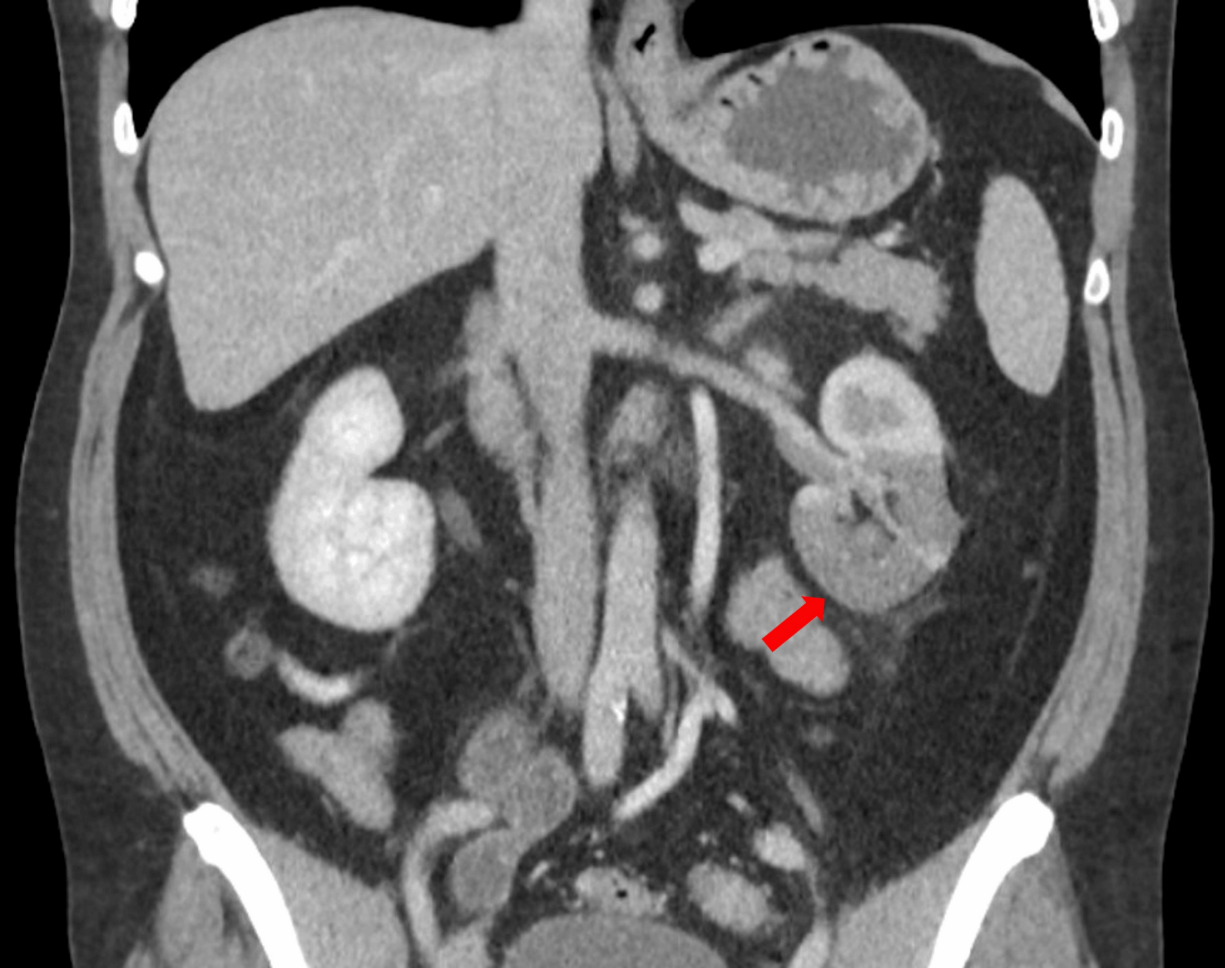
Computed tomography of the abdomen and pelvis revealing hypodense foci in the left kidney, suggesting parenchymal hypoperfusion in the medial and inferior portions (red arrow).
